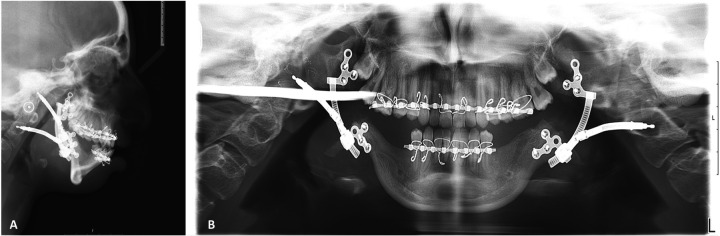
Post-activation radiography: (A) lateral cephalometry demonstrating overcorrected negative overjet, in close similarity to virtual planning (Figure 1C), improved position of pogonion, and increased airway. (B) Panoramic radiograph demonstrating the symmetry of bilateral elongation.
